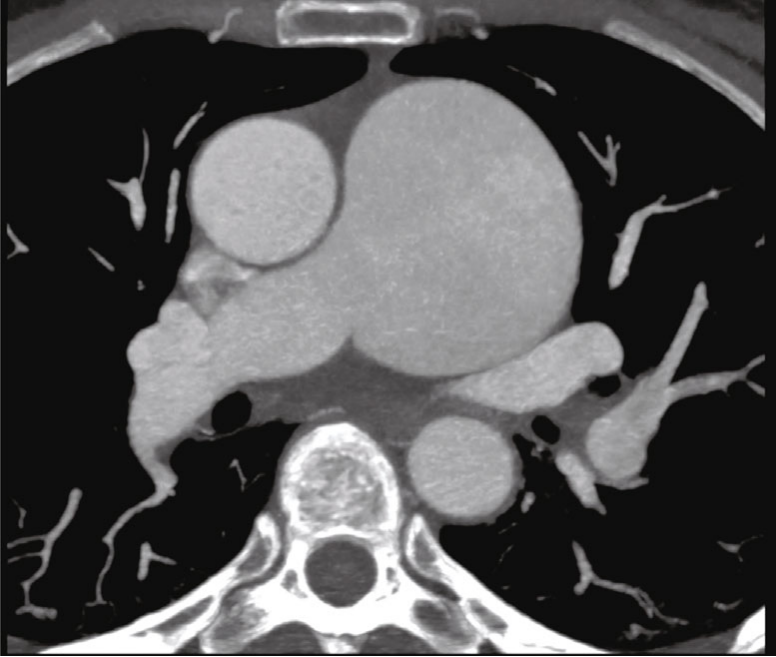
Cardiac CT axial images showing a 6.2 × 6.1 cm PAA.
